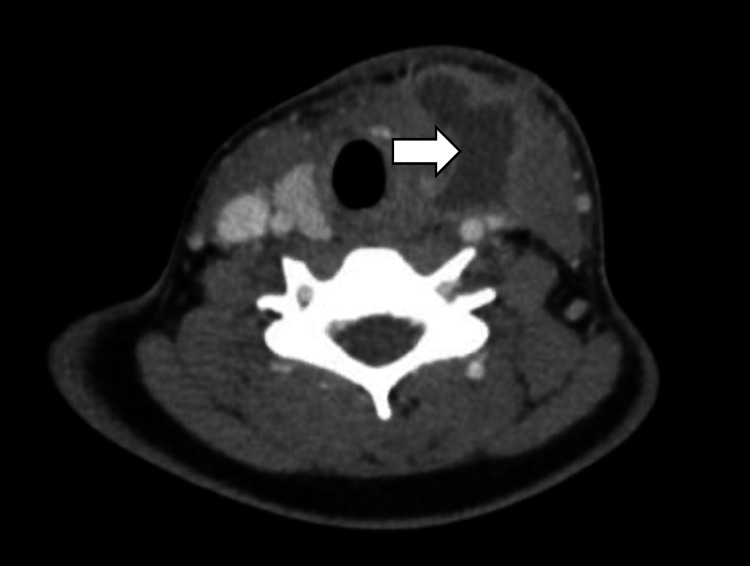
CT neck with contrast White arrow demonstrates the left fourth branchial cleft cyst tracking towards the left pyriform sinus.
